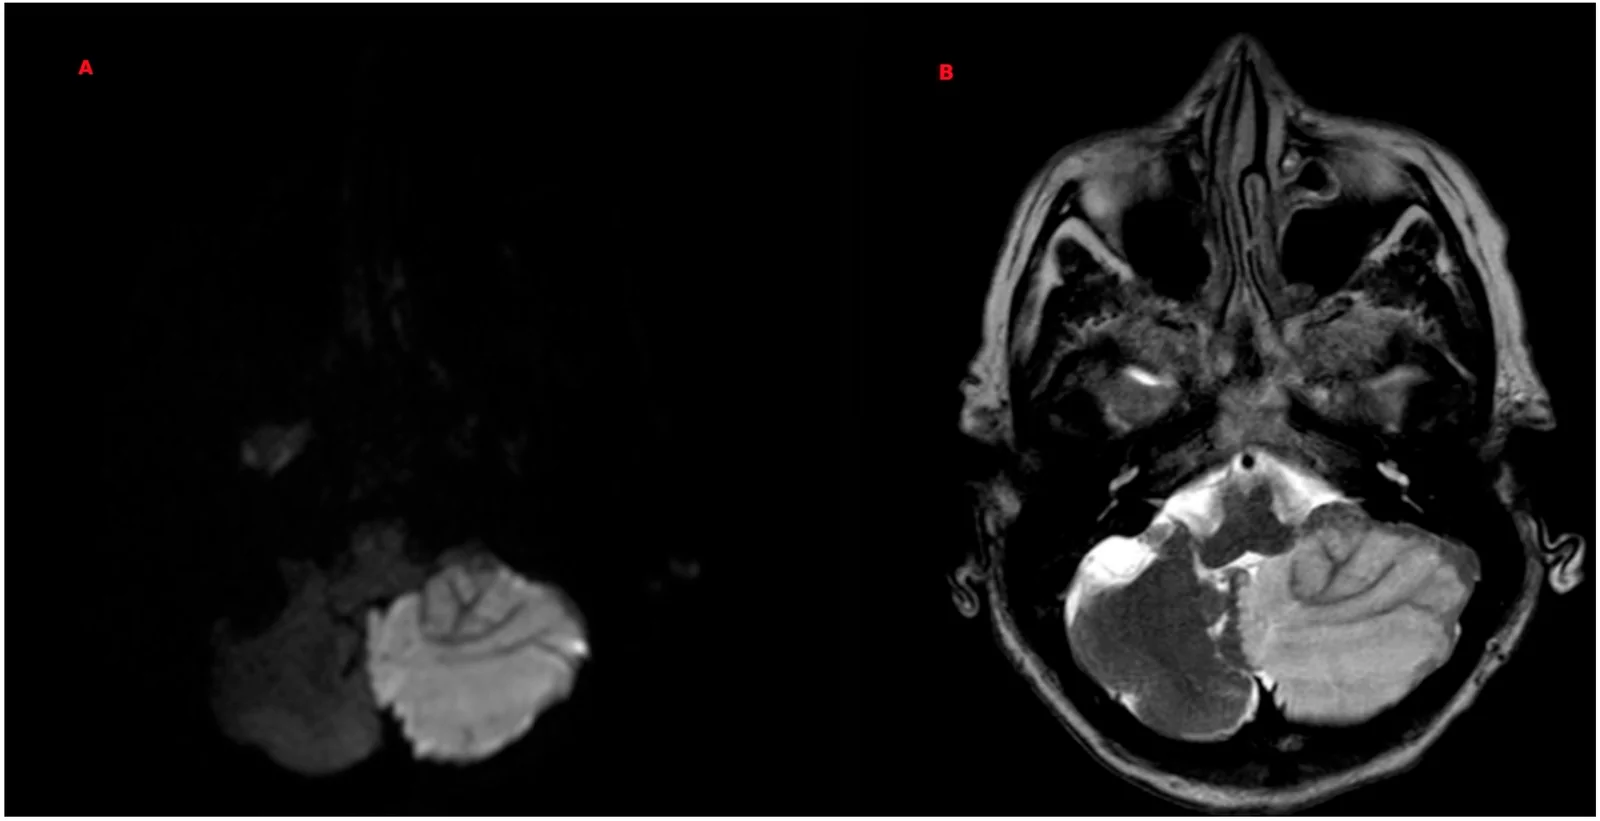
Acute left PICA-territory cerebellar infarction. (A) Axial diffusion-weighted MRI shows marked hyperintensity in the left cerebellar hemisphere, consistent with the reported acute infarction. (B) Axial T2-weighted MRI shows corresponding hyperintensity and edema. An apparent diffusion coefficient map is not displayed.
